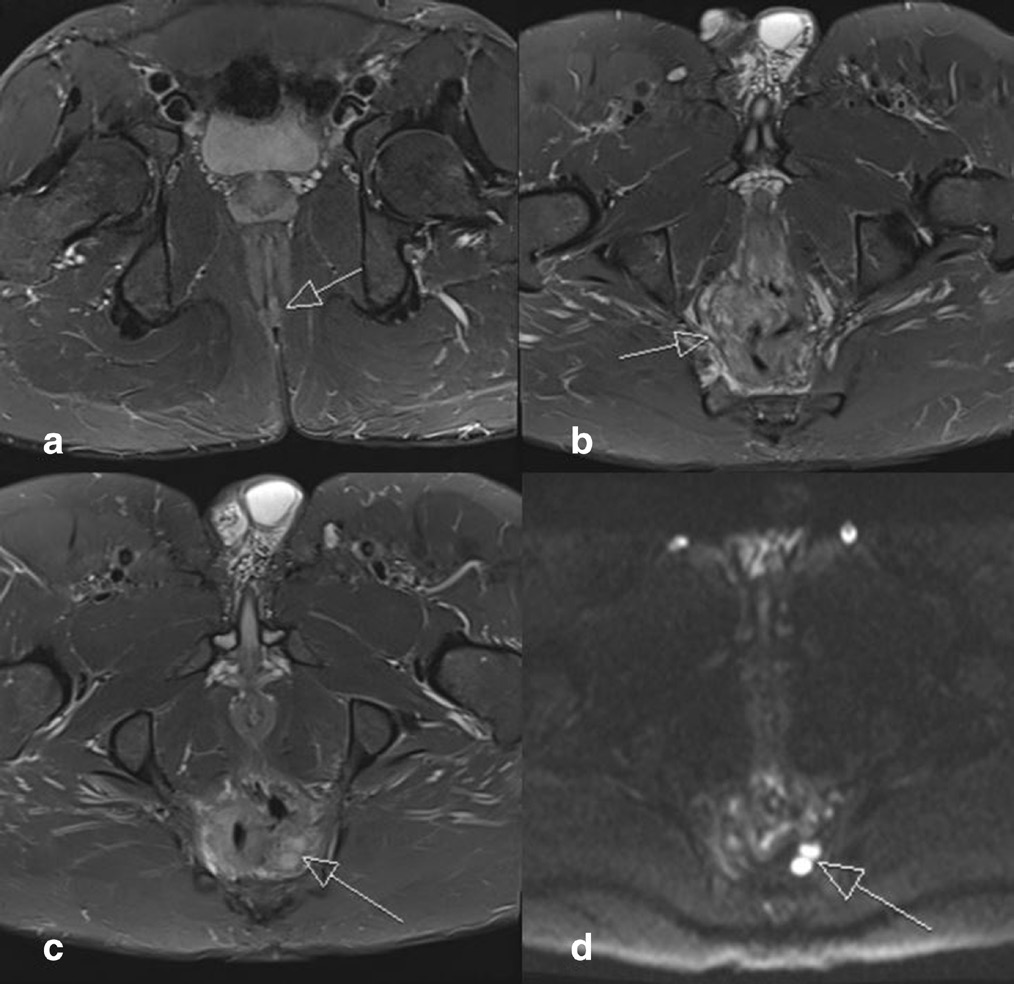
A. Axial STIR image demonstrates high signal around the anal verge at the perianal region in keeping with mild perianal inflammation (arrow). Figure 1B. Axial STIR image demonstrates high signal and thickening of the rectum in keeping with mild proctitis (arrow). Figure 1C. Axial STIR image demonstrates prominent mesorectal lymph nodes secondary to the adjacent proctitis. Figure 1D. Axial DWI image demonstrates corresponding restricted diffusion in the mesorectal lymph nodes.
